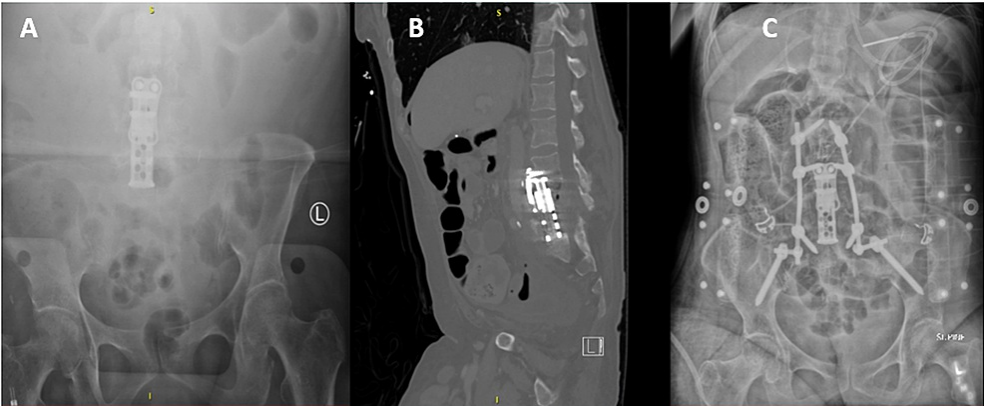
A) Post-operative abdominal supine radiograph in the AP projection  and B) Post-operative sagittal multiplanar reconstruction of the lumbar spine from an abdominal pelvic computed tomography demonstrating anterior stabilization of the spine using a K2M Capri Corpectomy cage and C) Post-operative abdominal supine AP radiograph demonstrating anterior and posterior stabilization
